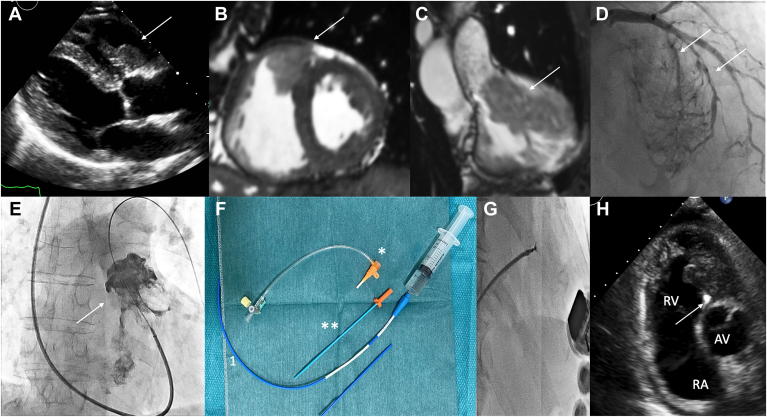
(A) Transthoracic echocardiography (TTE), end-diastolic parasternal long-axis view, arrow indicating right ventricular mass. (B) Axial short-axis cine magnetic resonance imaging, arrow indicating right ventricular outflow tract (RVOT) mass. (C) Sagittal cine magnetic resonance imaging, right ventricular outflow, arrow indicating RVOT mass. (D) Coronary angiography, left anterior oblique cranial, arrows indicating vascularization of the mass by 2 septal branches. (E) Angiography of the mass through a right internal jugular access, arrow indicating the RVOT pediculated mass. (F) 8-F (blue) 100-cm-long right coronary Judkins 4 Launcher (Medtronic) guiding catheter, which was shortened by cutting approximately 15 cm (shown by 1). We reconnected the distal and proximal portions of the guiding catheter using the distal part of a 7-F (orange) Terumo (Tokyo, Japan) introducer sheath Therefore, the proximal portion of the introducer sheath with the valve was cut, (indicated by asterisk). The dilator (indicated by double asterisk) of the introducer sheath was used to facilitate the reconnection of the 2 pieces of the guiding catheter using a portion of the introducer sheath (X). (G) Biopsy guided by fluoroscopy and TTE through a right femoral access using an 8-F regular 100-cm-long guiding catheter, which was initially too long to open a 104-cm-long Cordis bioptome. (H) TTE, parasternal short-axis view, arrow indicating the bioptome (hyperechoic) collecting pieces of the RVOT mass. AV, aortic valve; RA, right atrium; RV, right ventricle.
